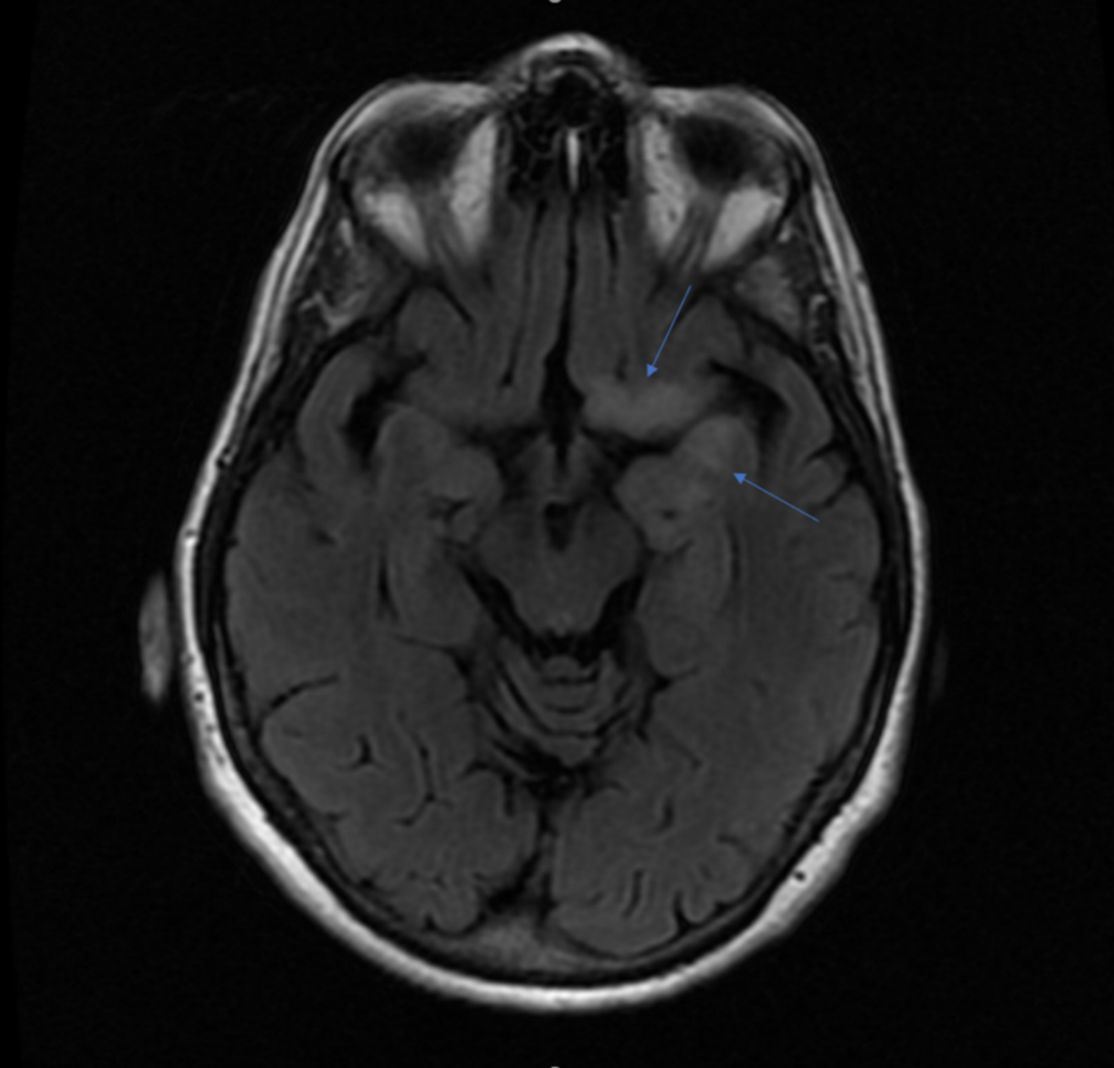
MRI of the brain revealing non-enhancing T2/fluid-attenuated inversion recovery (FLAIR) abnormalities affecting the area of the left frontal lobe, the left subcallosal region and the left mesial temporal lobe.
